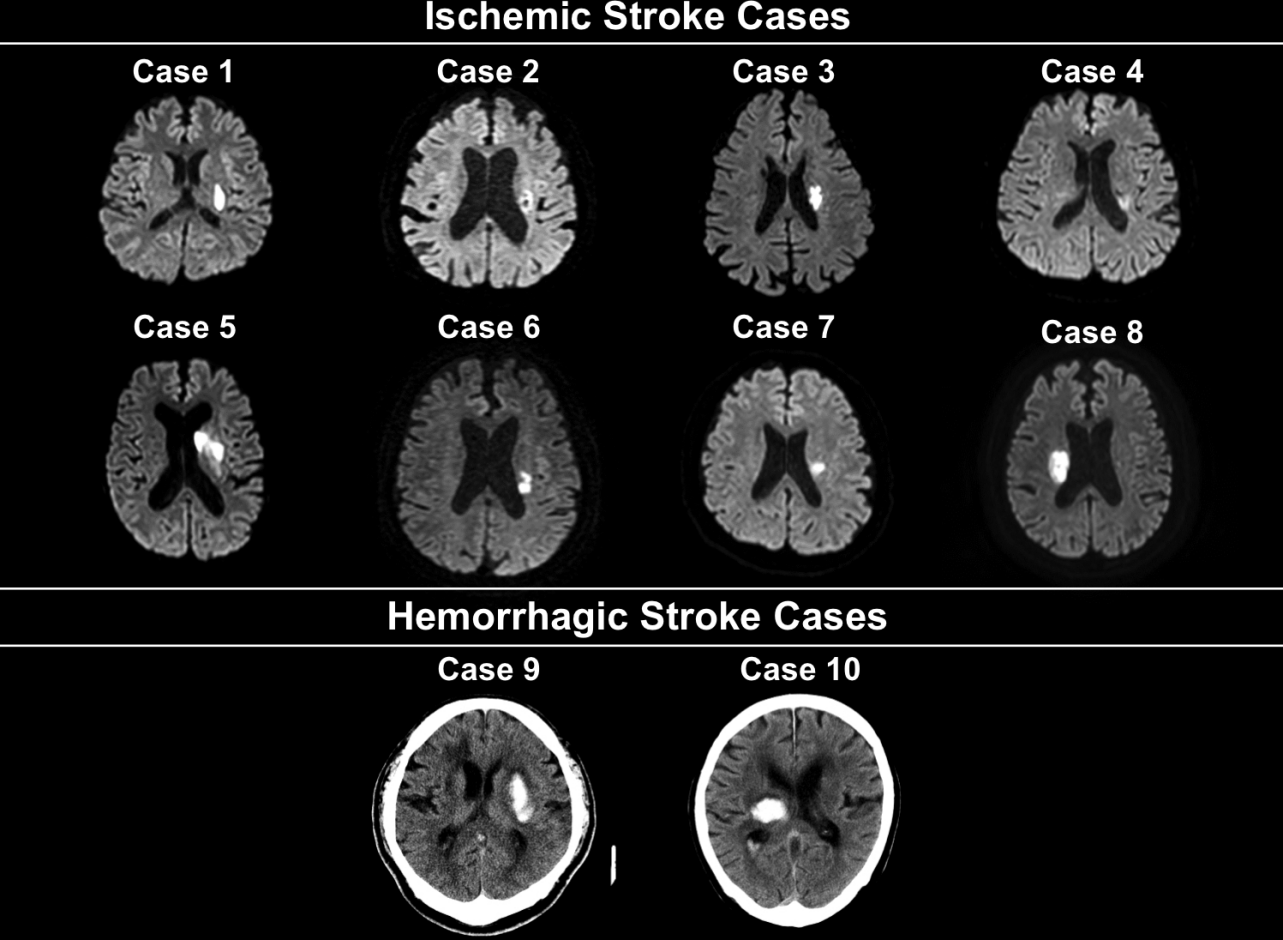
MRI/CT images presenting the stroke lesion of 10 study subjects. Diffusion-weighted images and CT images are presented to demonstrate the stroke lesions of ischemic and hemorrhagic stroke cases, respectively.
